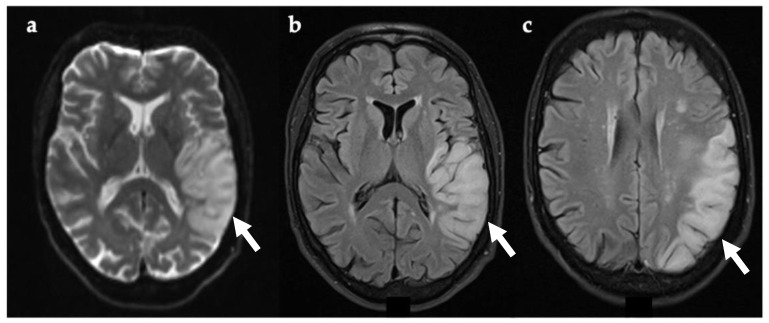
Axial brain MRI 24 h (a,b) and seven days (c) after symptom onset. Evidence of restricted diffusion in left temporal lobe (DWI) (a, white arrow). T2-FLAIR showing cortical and gyral swelling in left temporal lobe (b, white arrow). Image (c, white arrow) was taken seven days after symptom onset and shows extension of lesion on the left occipital lobe. Abbreviations: DWI, diffusion-weighted imaging; FLAIR, fluid attenuated inversion recovery imaging.
